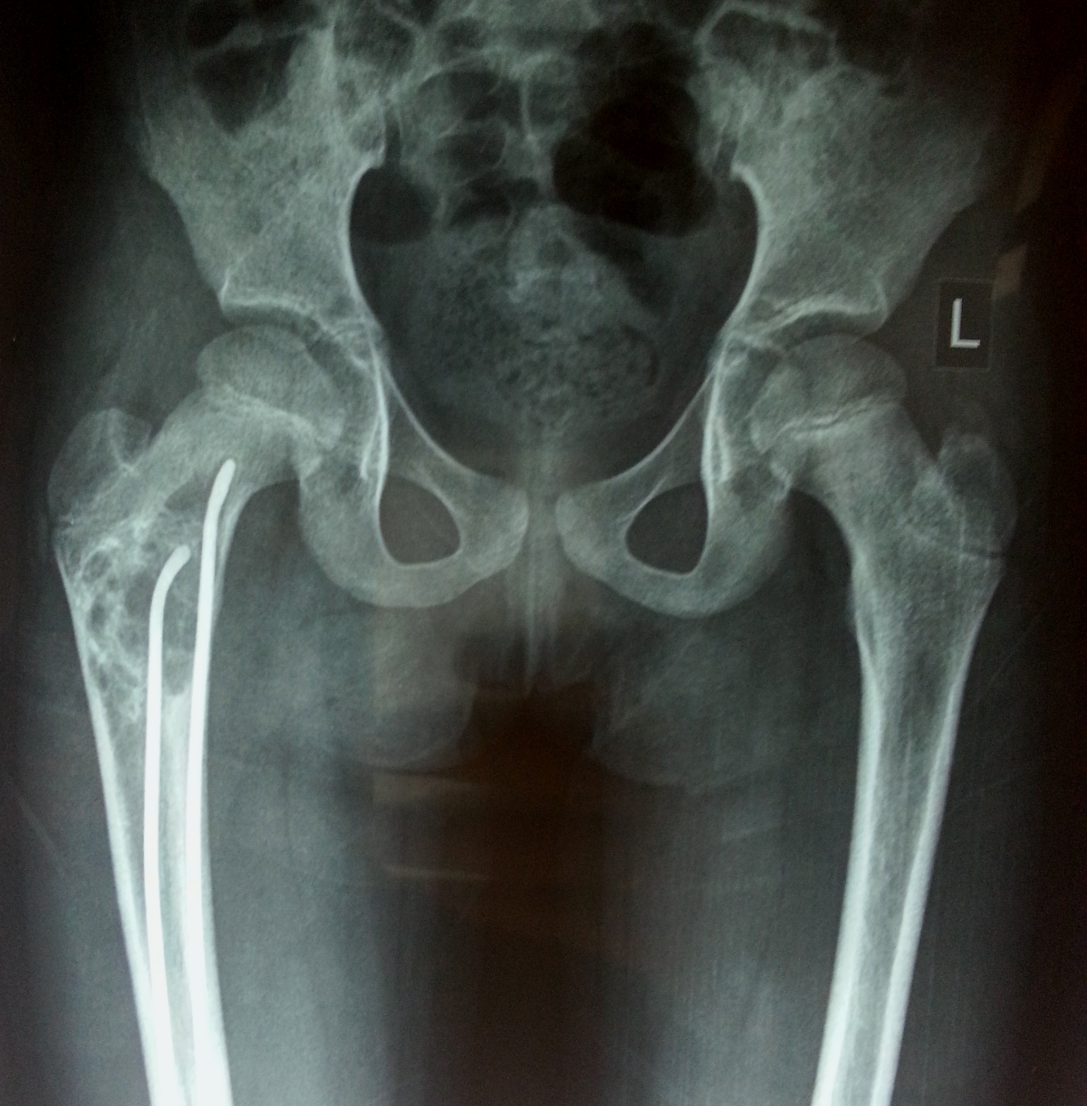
Follow-up radiograph at 13 months Shows recurrence of the lesion with ender’s nails in situ.
